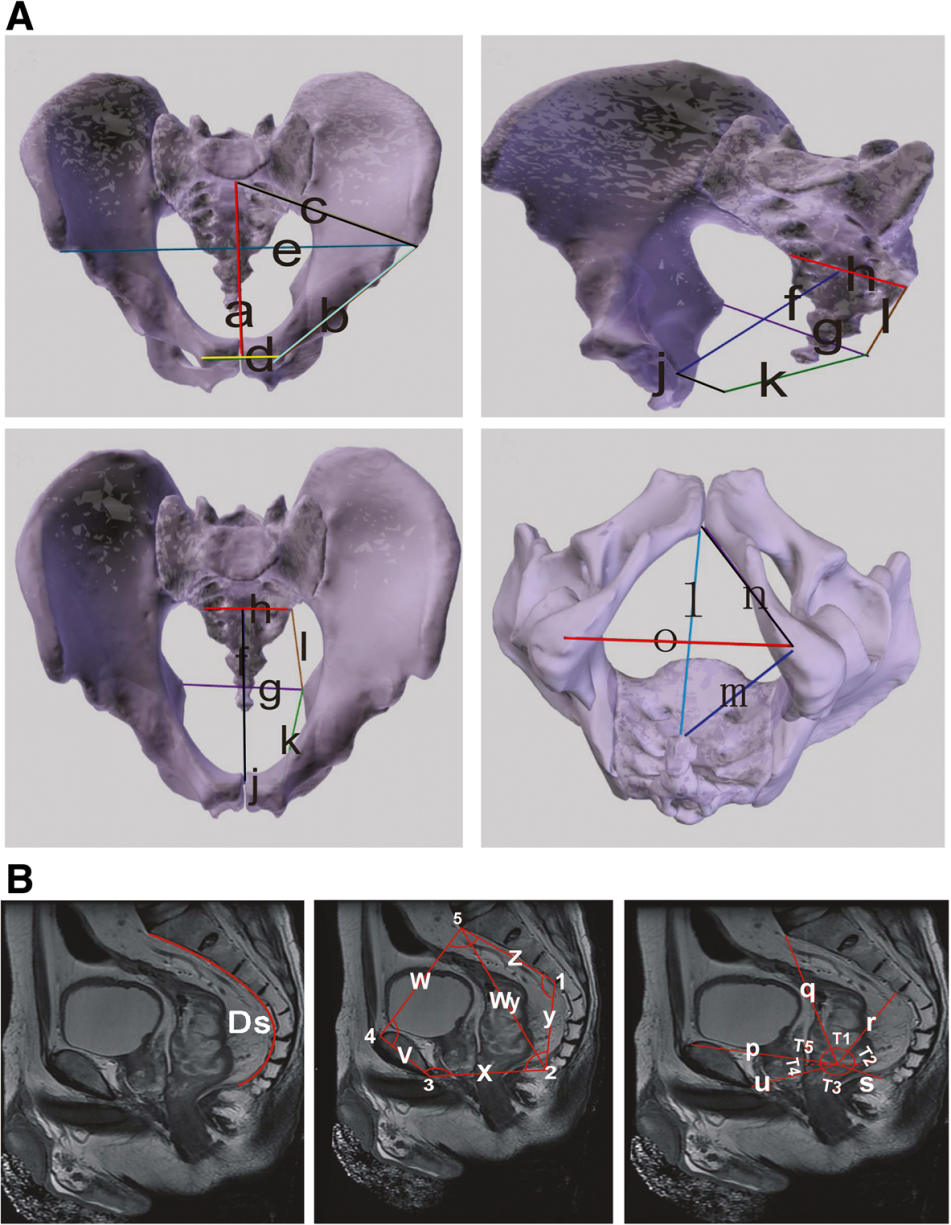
a Definition of pelvic parameters assayed by CT. Pelvic inlet plane: (a) anteroposterior diameter of entrance; (b) diameter between anterior superior iliac spine and pubic bone; (c) diameter between anterior superior iliac spine and sacrum; (d) superior border diameter of pubis tubercle; (e) anterior superior iliac spine diameter. Middle pelvic plane: (f) anteroposterior diameter of middle pelvis; (g) bispinous diameter; (h) S3 width; (i) diameter of S3 and ischial spine; (j) midline diameter of pubis; (k) diameter between pubis and spinal ischiadica. Pelvic outlet plane: (l) diameter between pubic bone and coccyx; (m) diameter between ischial tuberosity and coccyx; (n) diameter between pubic and ishial tuberosity; (o) diameter of ischial tuberosity. b Definition of pelvic parameters assayed by MRI. (v) Suprapubic diameter; (z) sacral S3; (Wy) sacrococcygeal diameter; (Ds) caudal arc length; (y) S3 tail; (q) sacral T; (r) T-S3; (s) T tail; (p) T suprapubic; (u) T subpubic; (1) the angle between the vertex of sacral promontory and the apex of the coccyx; (2) the angle between the lower edge of the third sacral bone and the lower margin of the pubic bone; (3) the angle between the apex of the coccyx and the upper margin of pubis; (4) the angle between the vertex of sacral promontory and the lower margin of the pubic bone; (5) the angle between the vertex of sacral promontory and the lower edge of the third sacral bone; (T1) the angle between the vertex of sacral promontory and the lower edge of the third sacral bone; (T2) the angle between the lower edge of the third sacral tubercle and the apex of the coccyx; (T3) the angle between the apex of the coccyx and the lower border of the pubic bone; (T4) the angle between the lower border and upper border of the pubic bone; (T5) the angle between the upper border of the pubic bone and the vertex of sacral promontory
